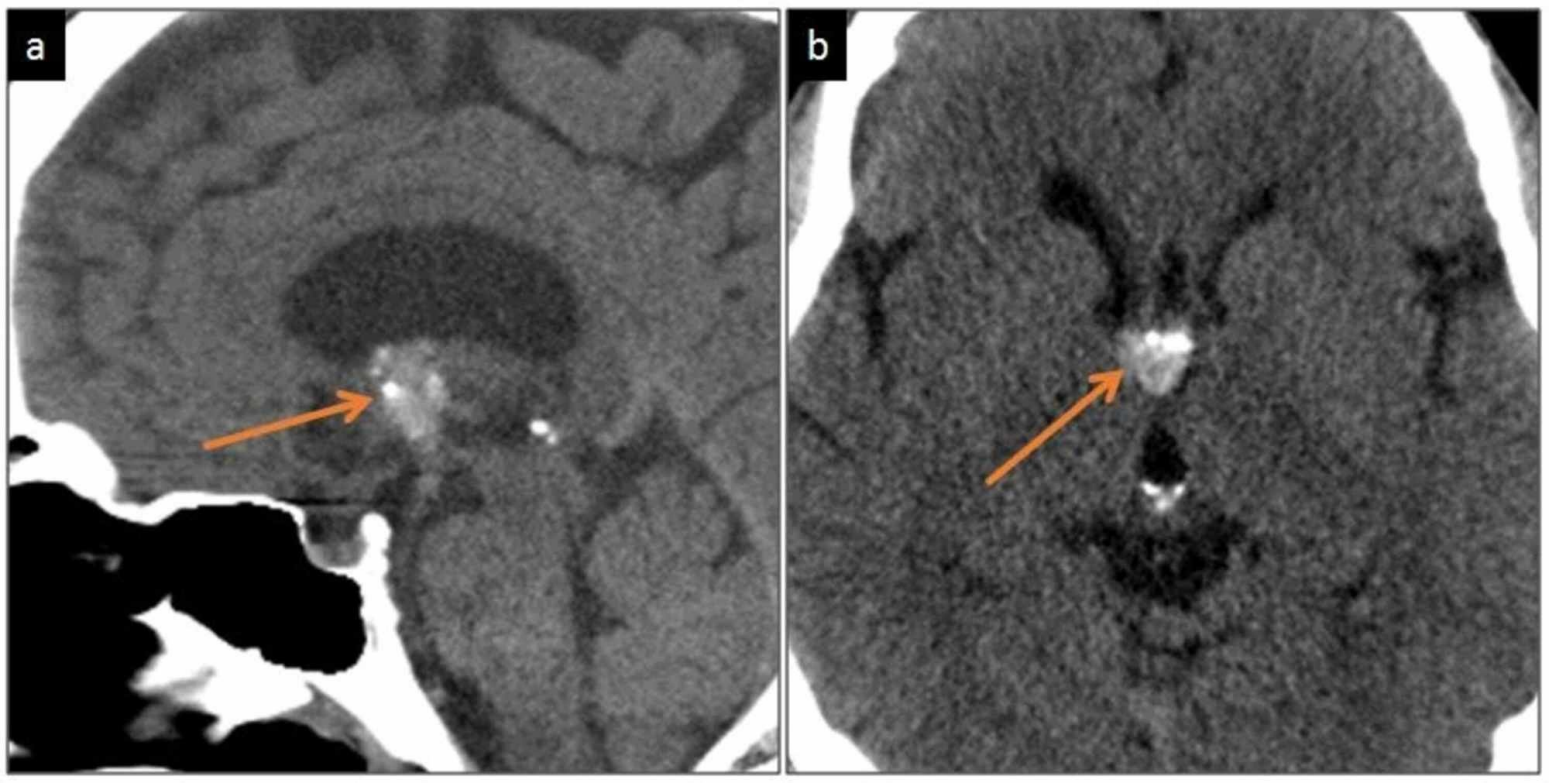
Unenhanced CT brain Sagittal (a) and axial (b) images demonstrate a hyperdense lesion (orange arrows) at the anterior third ventricle, extending to the foramen of Monro and protruding into the anterior horn of right lateral ventricle. It contains tiny calcifications. The right lateral ventricle is mildly dilated
